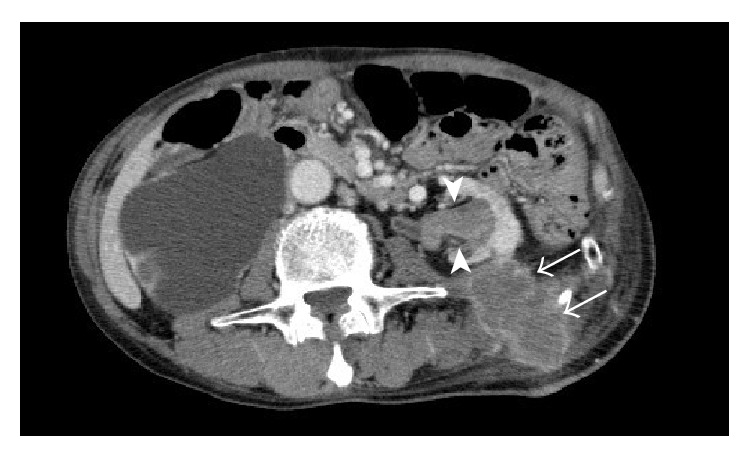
Computed tomography (CT) of the tumors. Abdominal CT scan showing a tumor in the left pelvis (arrowhead) and a soft tissue mass along the former nephrostomy tract (arrow).
